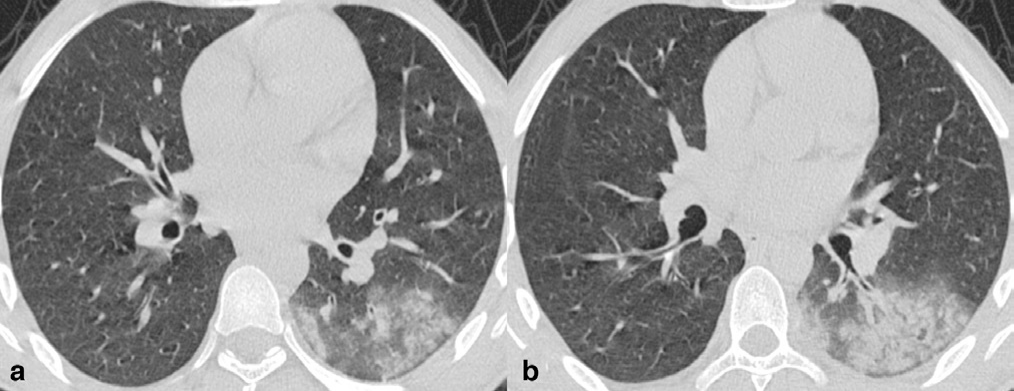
Axialchest CT images in lung window settings of a 29-year-old COVID-19 asymptomaticmale patient showing a GGO with few areas of consolidation in superior segmentof left lower lobe in subpleural location (A). Follow-up CT obtained after agap of 5 days reveals an increase in the size and density of the opacity withtransformation into a frank consolidation (B).
